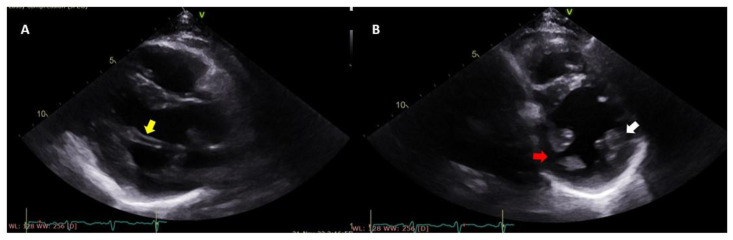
(A) PPM in parasternal long axis view (yellow arrow) in a patient with mitral valve prolapse. (B) Parasternal mid-ventricular short axis view at the level of PMs. Notice that PPM has two heads (red arrow), while APM has one (white arrow). (PPM: postero-medial papillary muscle; APM: antero-lateral papillary muscle, PM: papillary muscle).
